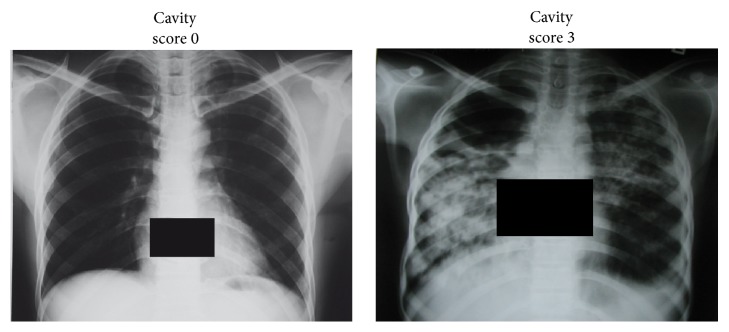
Posterior-anterior chest X-ray of TB cases. Exemplary cavity score 0 (no cavities), cavity score 3 (extended, large cavities) scored blinded by an experienced radiologist, cross-checked by an experienced physician.
